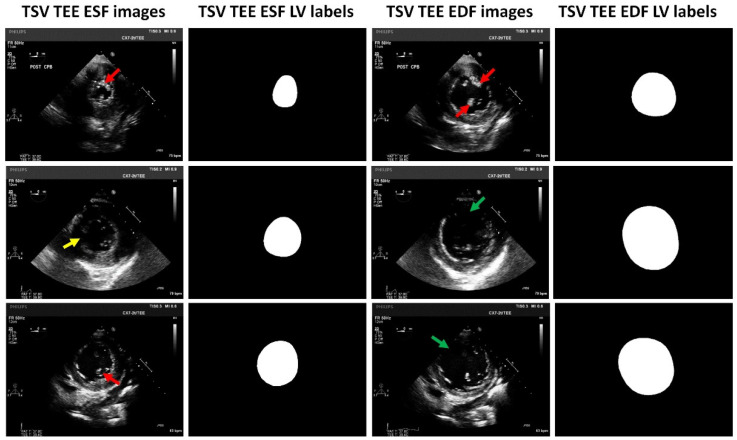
Representative TSV TEE images and corresponding LV labels. Red arrows indicate the papillary muscle. Yellow arrows indicate mild LV boundary missing. Green arrows indicate moderate LV boundary missing. LV: left ventricle; TSV: transgastric short-axis view; TEE: transesophageal echocardiography; ESF: end-systolic frame; EDF: end-diastolic frame.
